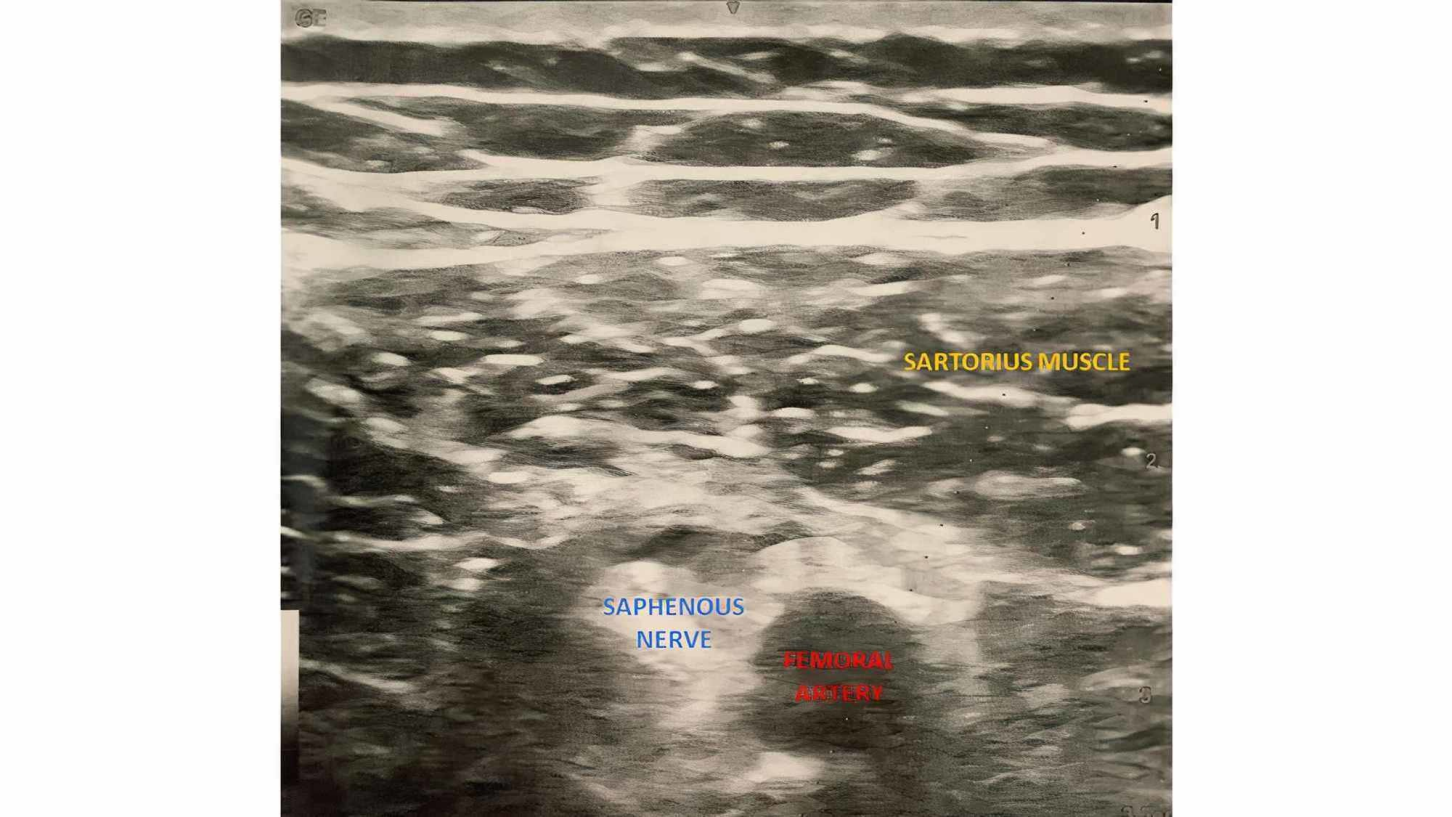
Gray-scale ultrasound with a linear probe showing the subsartorial space at the midthigh level. The sartorius muscle, saphenous nerve, and femoral artery can be seen.
